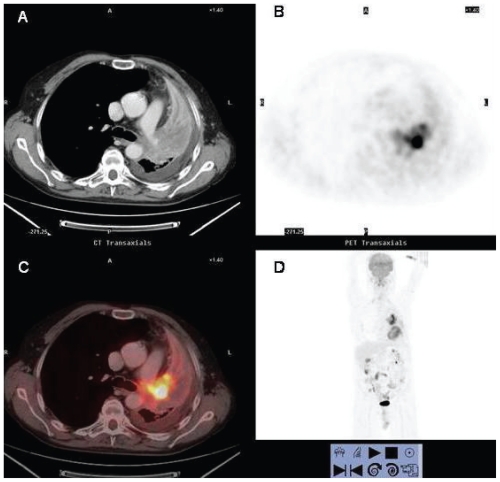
58 year-old man who underwent contrast-enhanced integrated ^18^FDG PET/CT for staging of newly diagnosed squamous carcinoma. Axial contrast-enhanced CT (A), axial (B) and coronal (D) PET and fused enhanced ^18^FDG PET/CT (C) images. Integrated enhanced ^18^FDG PET/CT (C) depicts the left upper lobe central ^18^FDG avid mass within the non-hypermetabolic collapsed lung parenchyma and allows differentiation between tumor and surrounding post-obstructive atelectasis. Thanks to the contrast-enhanced CT component of ^18^FDG PET/CT it is possible to delineate infiltration of the mediastinum and left pulmonary artery, classifying the tumor as a T4 stage. A left pleural effusion without ^18^FDG uptake is also noted. Accurate delineation of the tumor is important for radiation therapy planning.
